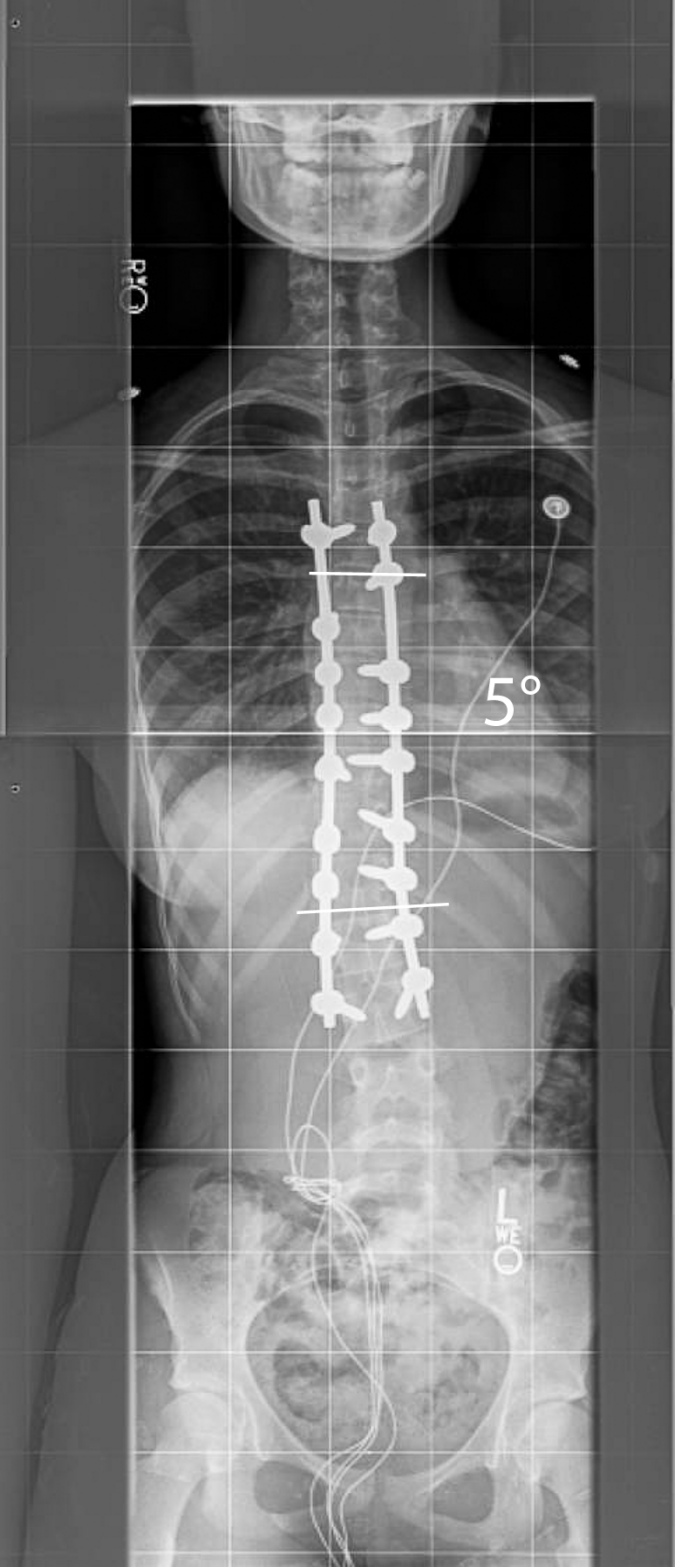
"Patient 2 - Postoperative anteroposterior and lateral radiographs." Postoperative images of the patient in Figure 13. A slightly different instrumentation pattern has been used (3-3-4 levels have been instrumented per incision). A good correction in both planes has been obtained, the shoulders are nearly level and the patient is balanced in the coronal plane.
